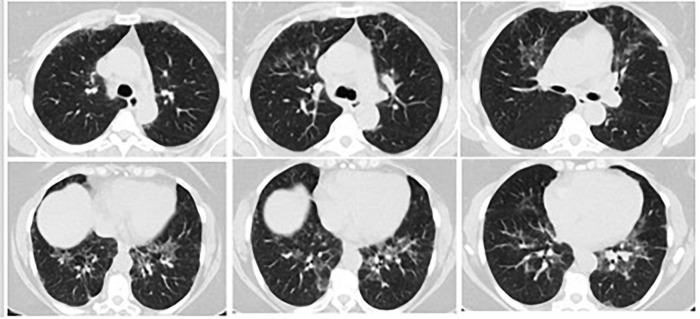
Three months later, on her repeated HRCT, bilateral peripheral peribronchovascular fibrotic reticulations in upper and lower lung lobes and increased areas of ground-glass opacities with pleural thickening around right posterior lung lobe were observed.
